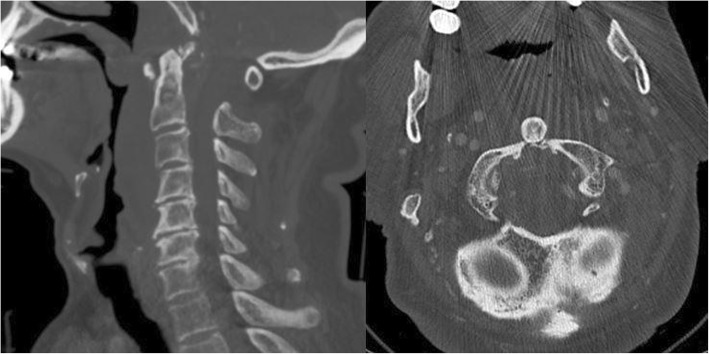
Pre-reduction mid-sagittal and axial CT slices of the cervical spine
